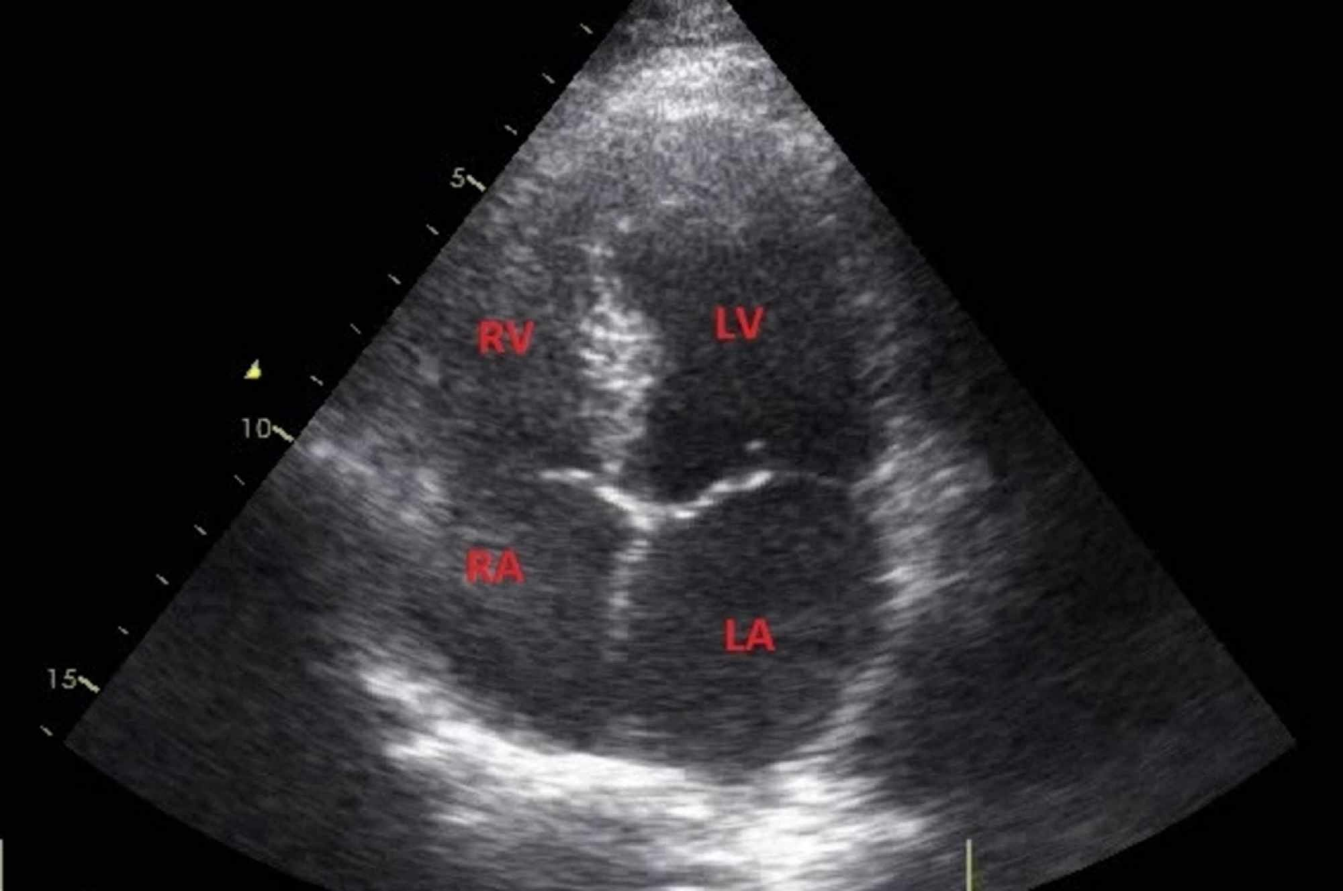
Transthoracic echocardiogram This is an apical four-chamber view of a normal transthoracic echocardiogram of the heart RA, right atrium; RV, right ventricle; LA, left atrium; LV, left ventricle
